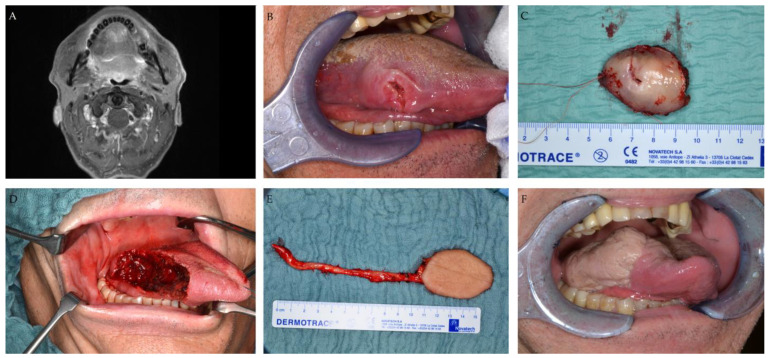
Patient with squamous cell carcinoma of the lateral tongue on the right side. (A) MRI scan (T1 sequence) depicting the tumor on the right side of the oral tongue. (B) Intraoperative image depicting the tumor. (C) Tumor after surgical excision. (D) Intraoral defect situation after tumor resection. (E) Harvested radial forearm flap. (F) Postoperative intraoral situation after tumor resection and reconstruction with radial forearm flap.
